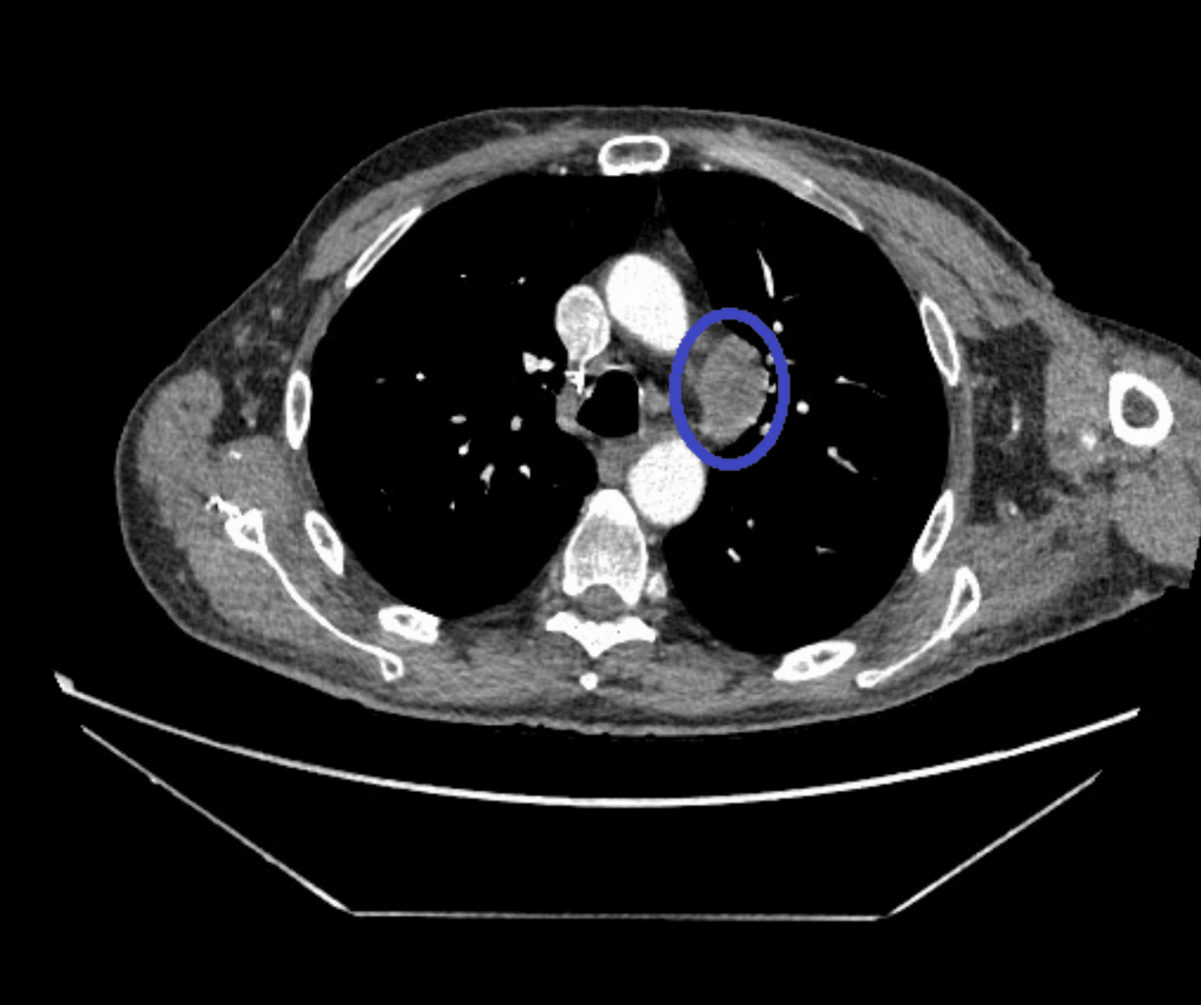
CT chest revealing a prominent perivascular lymph node in the aortopulmonary window CT: computed tomography
